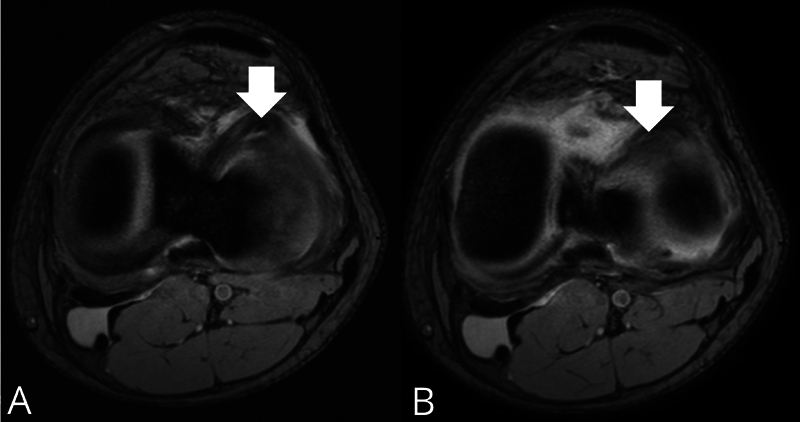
Preoperative MRI scan of a 17 year-old male subject with a surgically-reported bucket-handle tear of the lateral meniscus of the left knee. (
